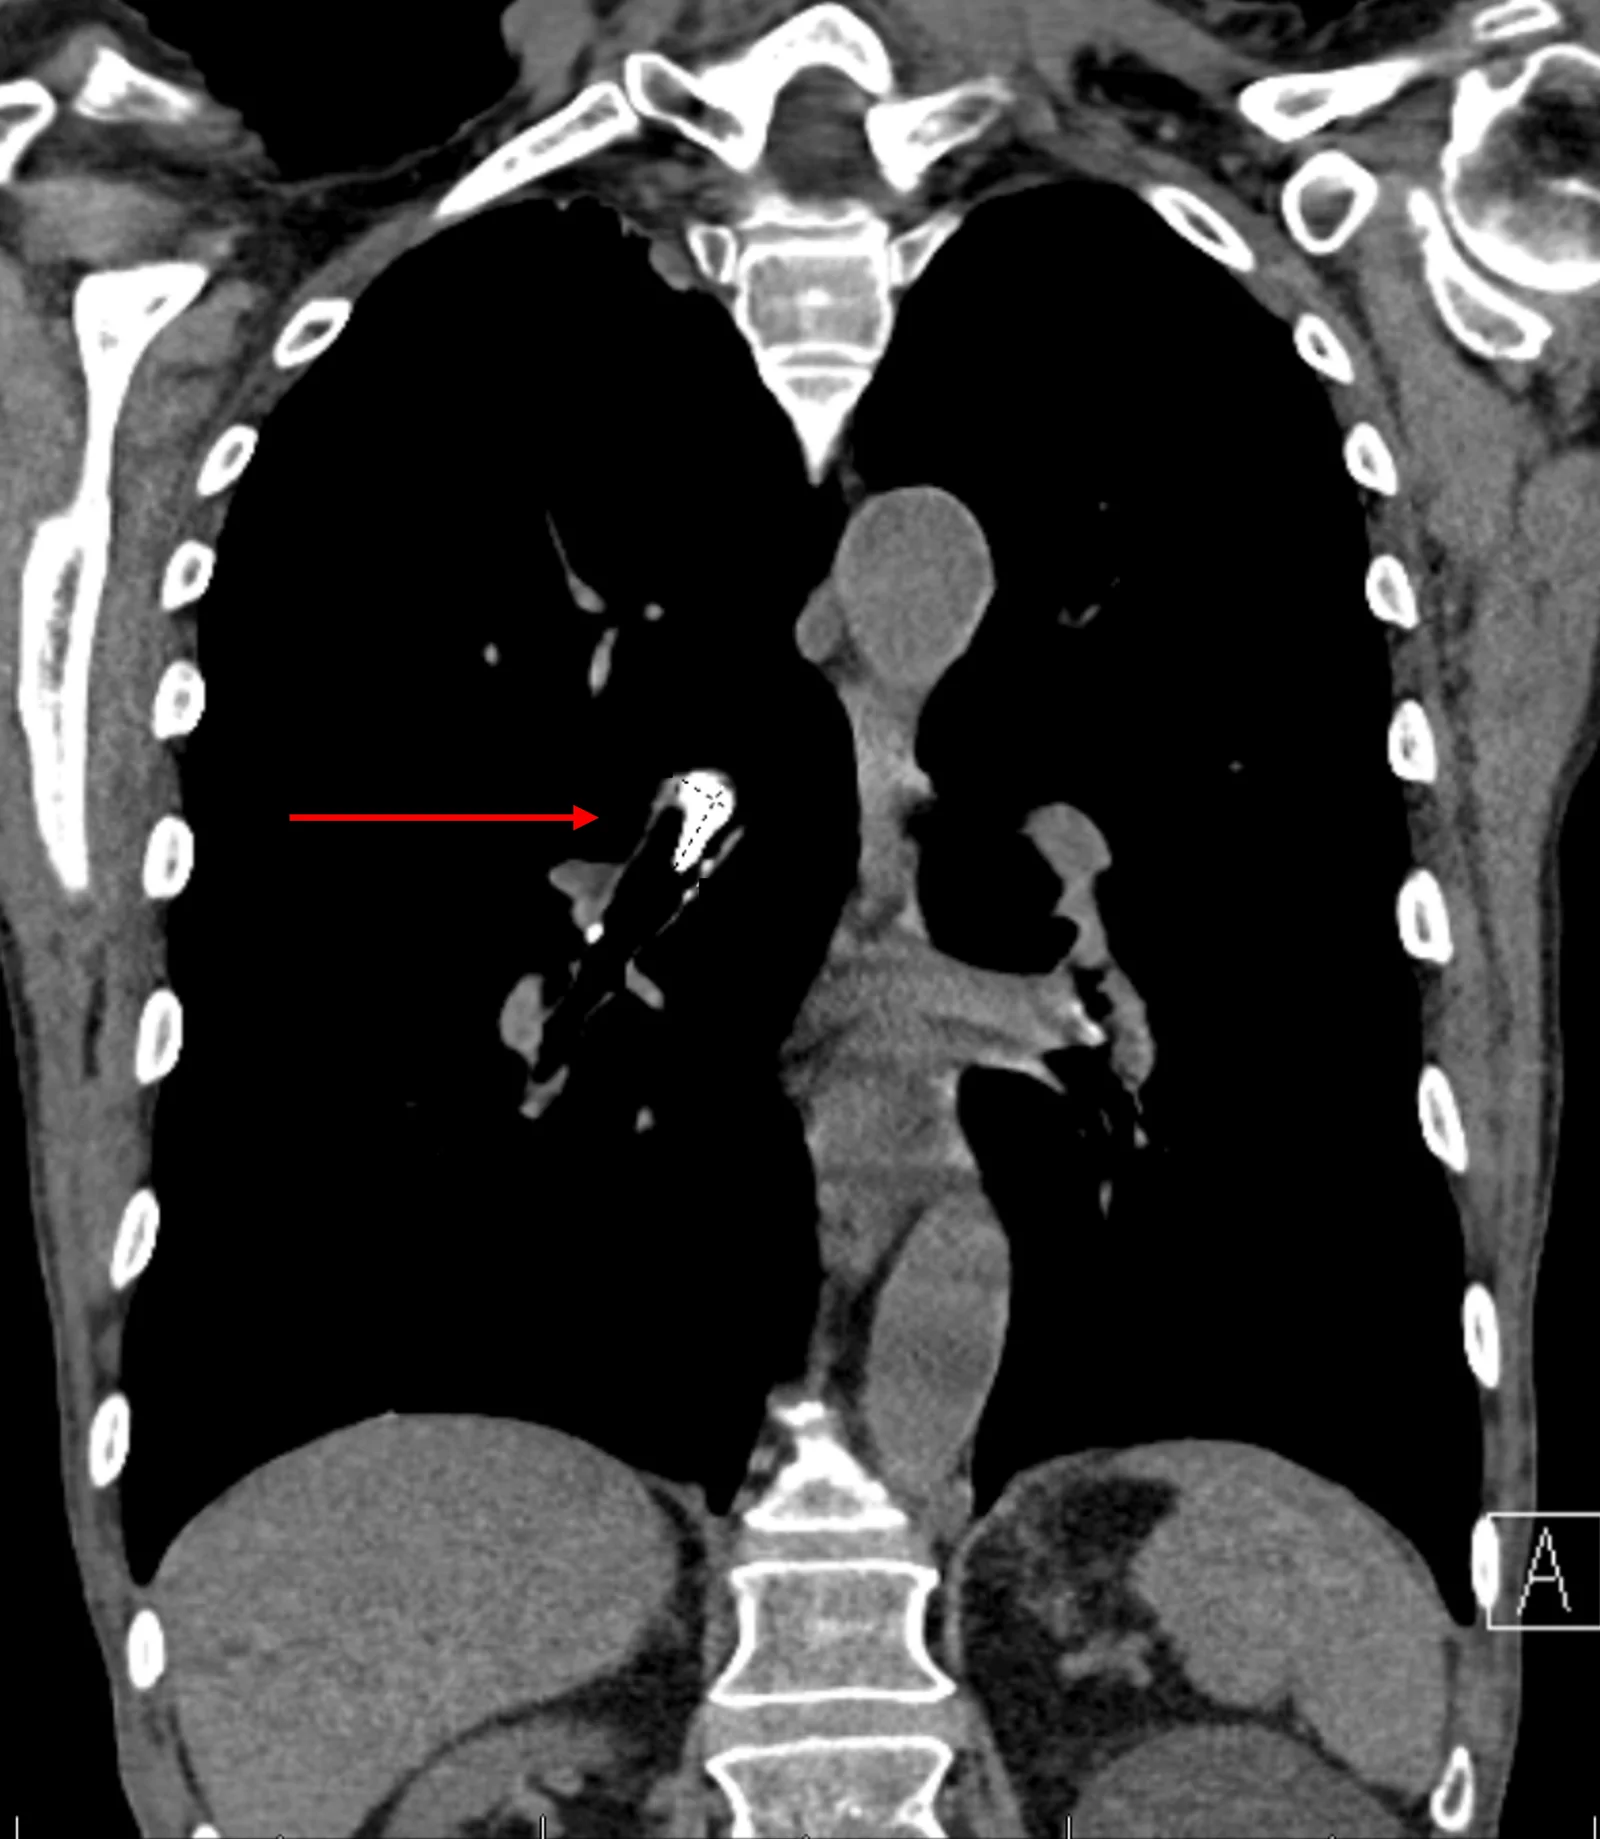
Contrast-enhanced coronal CT of the chest demonstrating a radiopaque aspirated molar (red arrow) lodged within the proximal right bronchus intermedius CT: computed tomography
